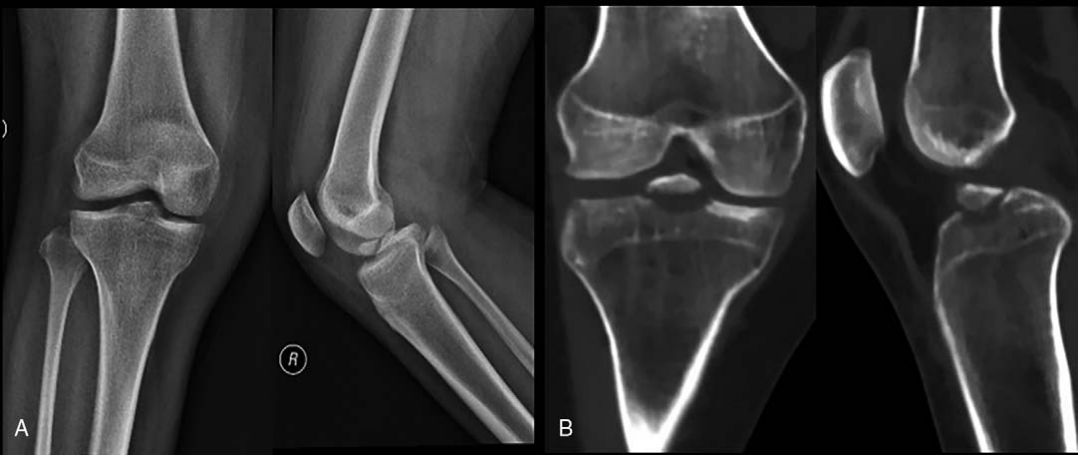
Preoperative radiograph (A) and CT (B) findings for a type III typical tibial eminence fracture according to modified Meyers-McKeever classification.
